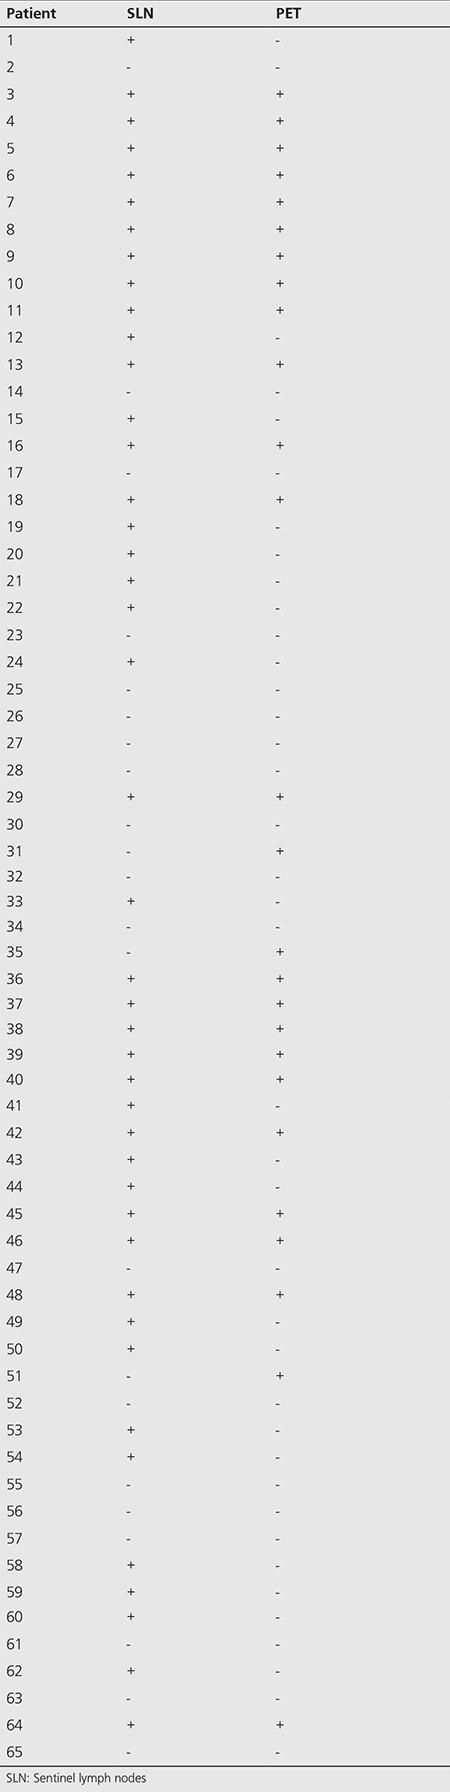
Sentinel lymph nodes biopsy results and PET/CT results of all patients
